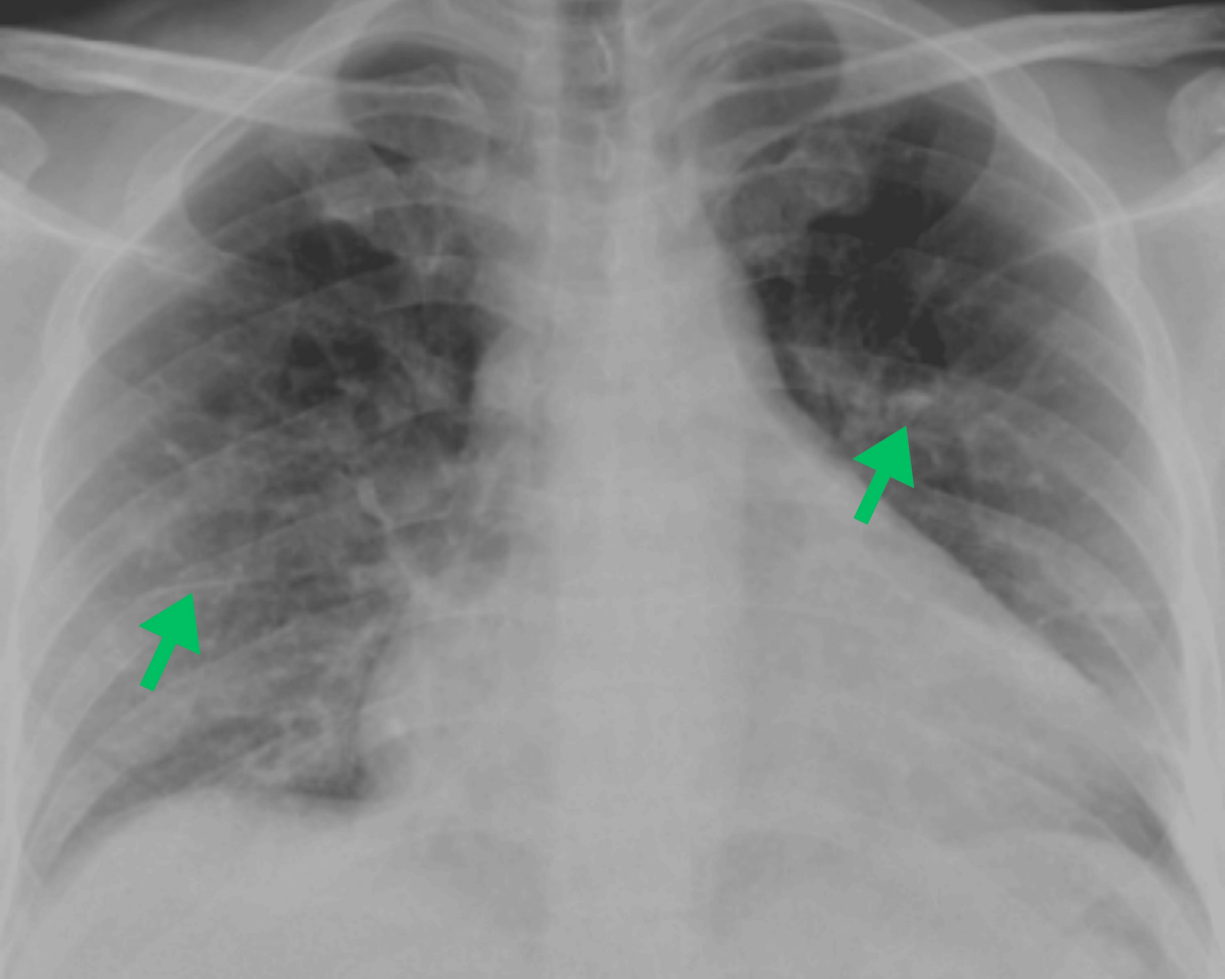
Follow-up chest radiograph at four months of treatment demonstrating marked improvement in bilateral pulmonary opacities and reduction of perihilar vascular congestion.
